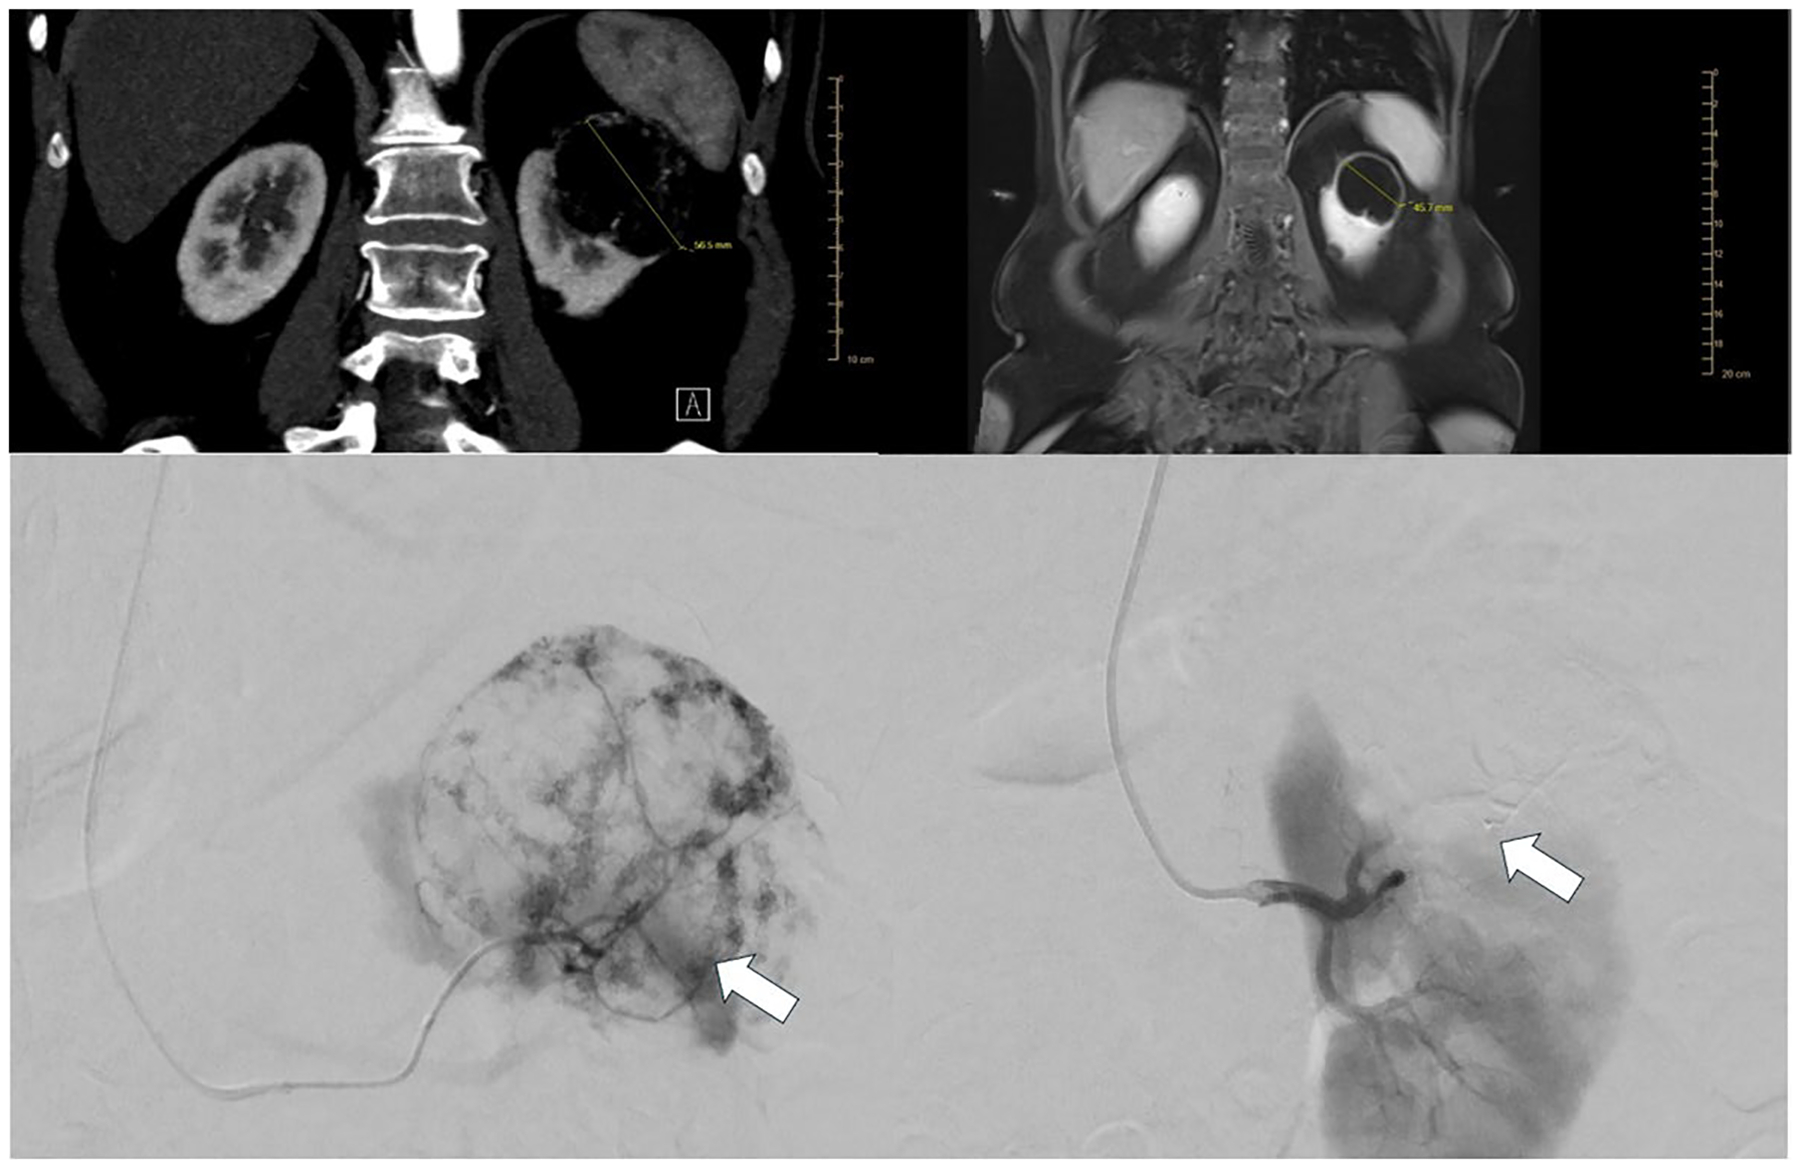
A 65-year-old female renal AML (A) Coronal contrast-enhanced CT scan shows a 5.7-cm enhancing renal mass in the upper pole of the left kidney (B) MRI at 3 months post-embolization demonstrating tumor diameter reduction of 1 cm. C Selective left renal arteriogram demonstrating a large hypervascular renal cell carcinoma (arrow) before embolization. D Microcatheter-mediated embolization of the hypervascular mass using Ta-OCE. Note the decreased regions of tumor vascularity (arrow)
